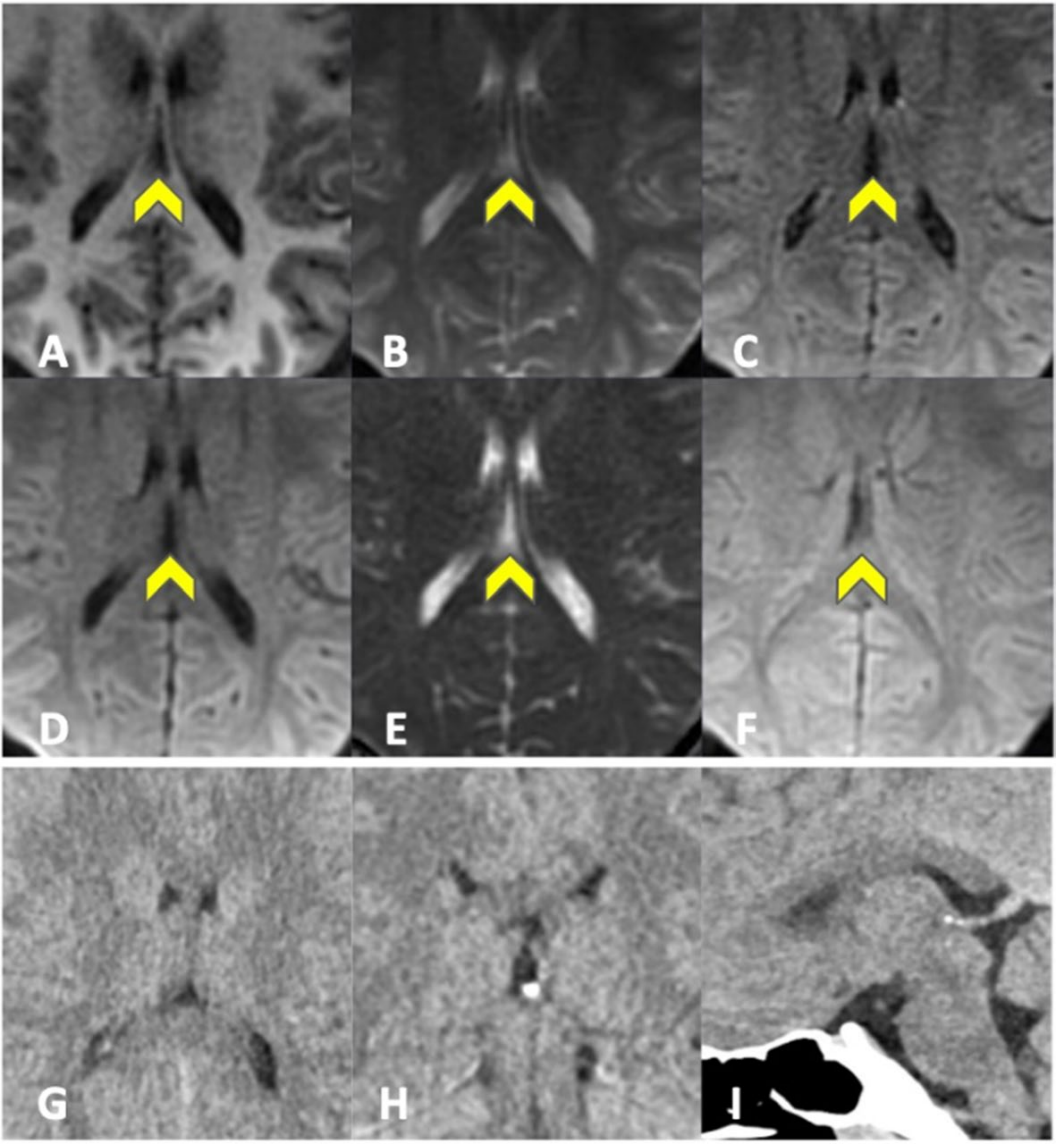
ID3. Midline CSF-filled cavities (yellow head arrow), visible only on EPIMix (side-by-side consensus). T1-FLAIR (A), T2 (B), T2-FLAIR (C), DWI (D), ADC (E), T2* (F) EPIMIX images, and axial (G), coronal (H), sagittal (I) soft tissue window CT images for reference
